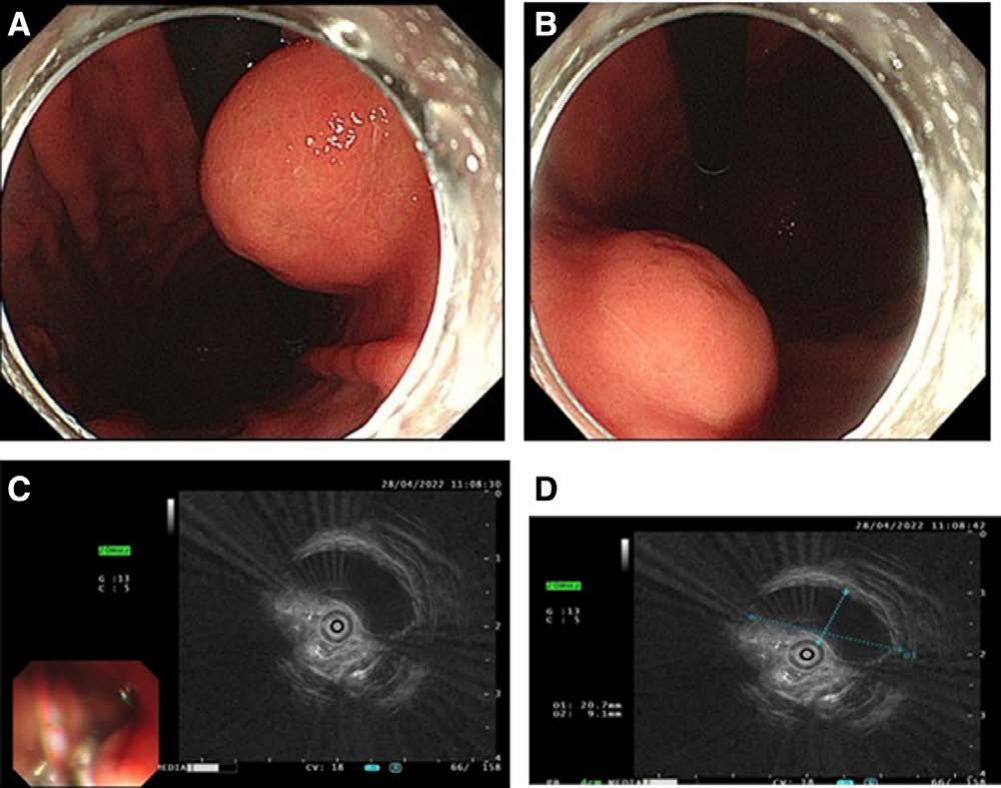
Endoscopic findings. (A and B) A hemispherical smooth submucosal mass with a central depression was observed on white light endoscopy. (C and D) Endoscopic ultrasonography showed a hypoechoic mass with uniform internal echo originating from the muscularis propria layer.
